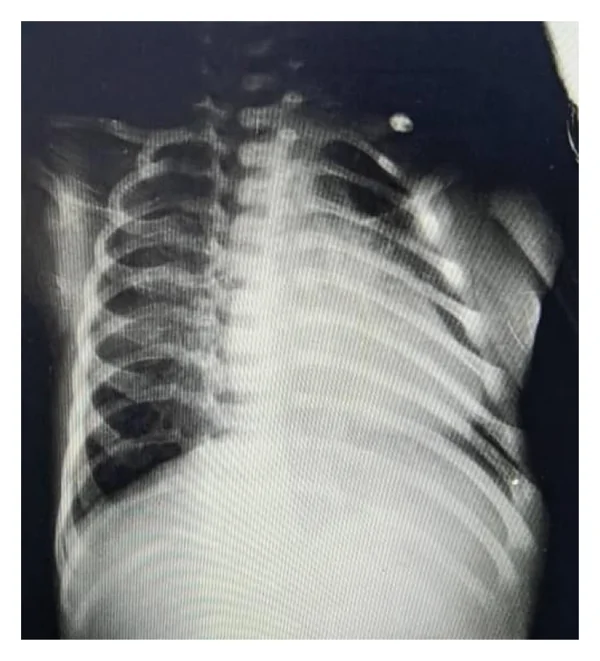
Chest radiography demonstrating mild bilateral pulmonary infiltrates.
